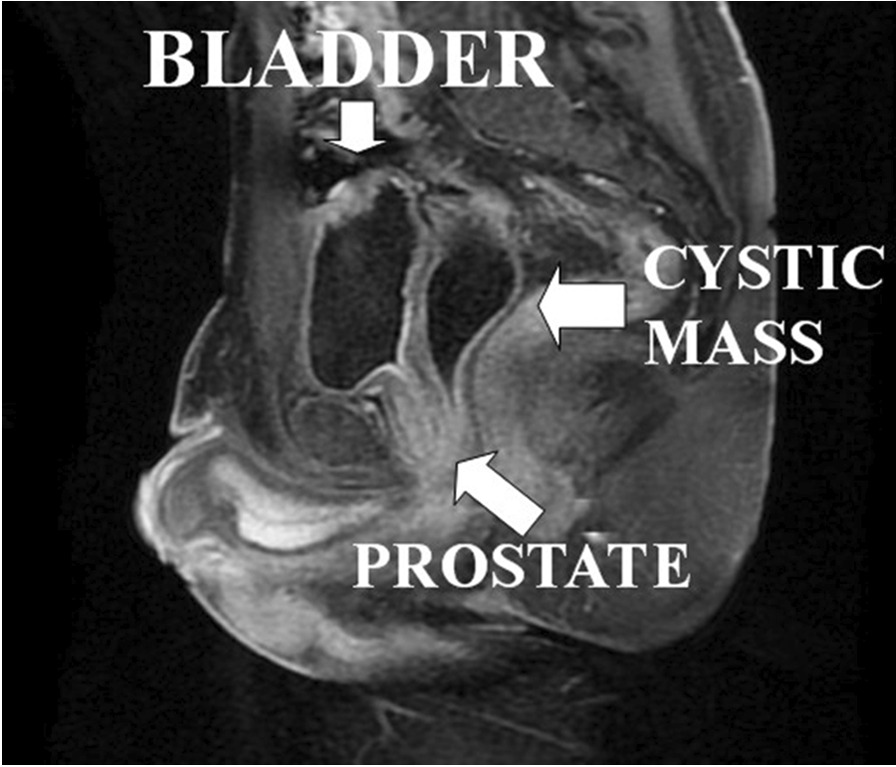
MRI shows the communication between the prostatic urethra and the cystic mass
